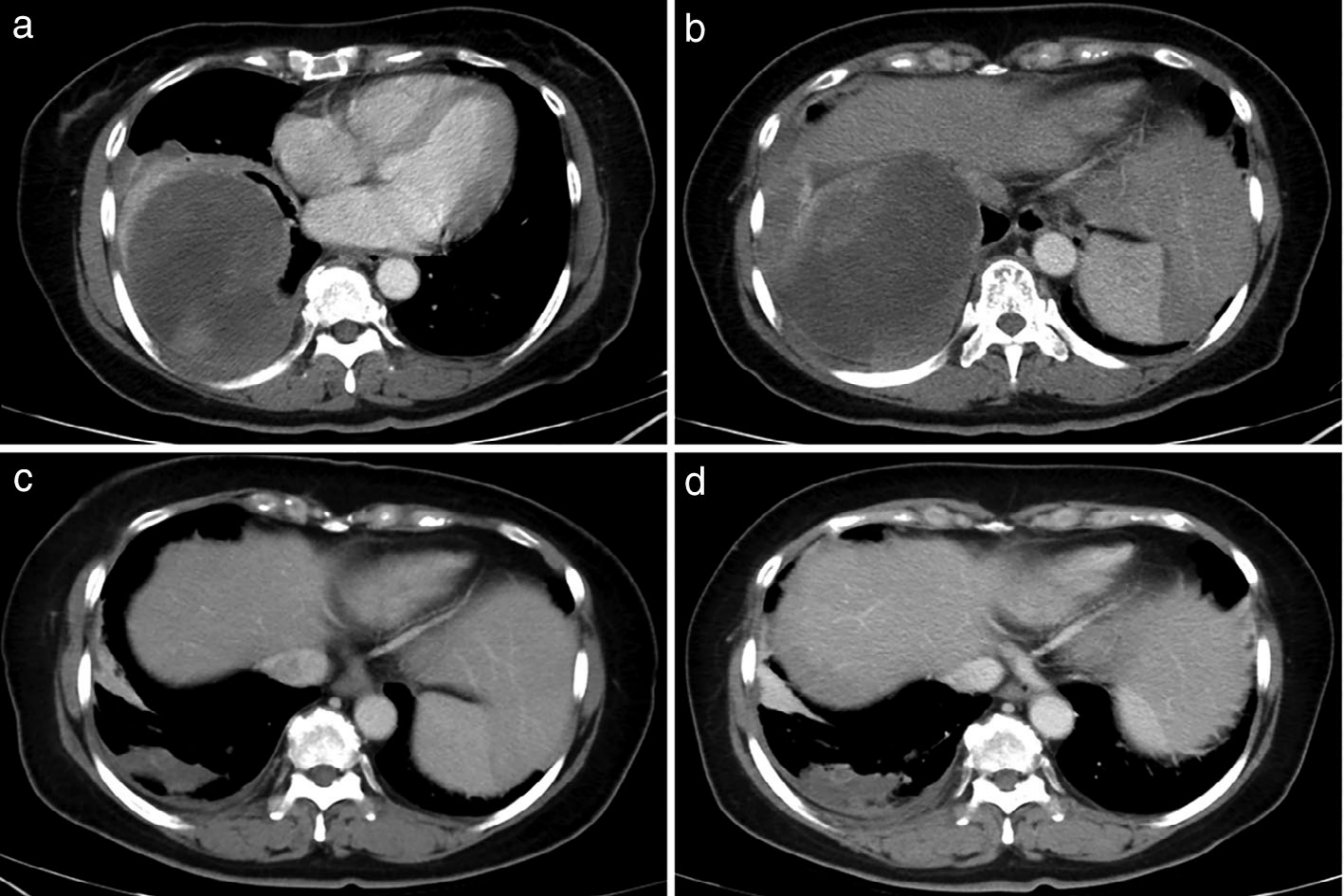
Enhanced CT scan. (a and b) A clear boundary appeared and heterogeneous enhanced soft tissue mass in the right pleura, with the maximum diameter 10.9 × 9.5 cm before chemotherapy. (c) The lesion shrunk to 5.2 × 2.0 cm after eight cycles chemotherapy. (d) The tumor was stable one month after RT.
